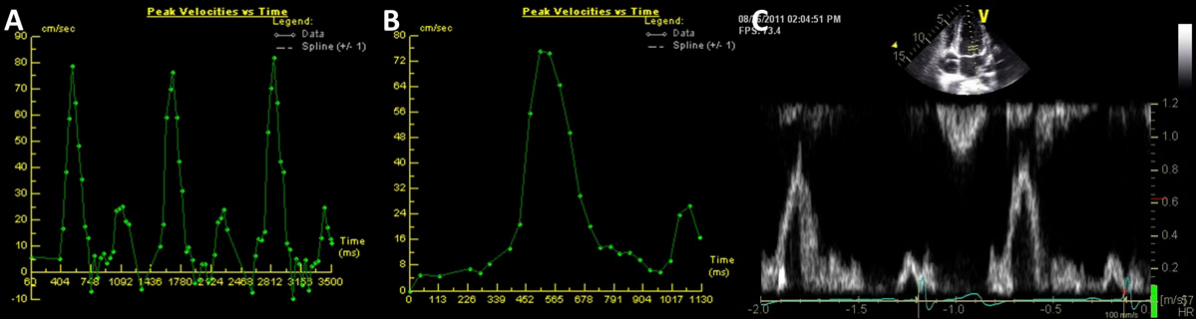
Mitral inflow velocities in one volunteer by all three techniques: (A) real-time phase contrast imaging (mean E, A, and E/A were 78cm/s, 25cm/s, and 3.1), (B) segmented phase contrast imaging (mean E, A, and E/A were 75cm/s, 27cm/s, and 2.8), and (C) Doppler echocardiography (mean E, A, and E/A were 77cm/s, 26cm/s, and 3.0).
